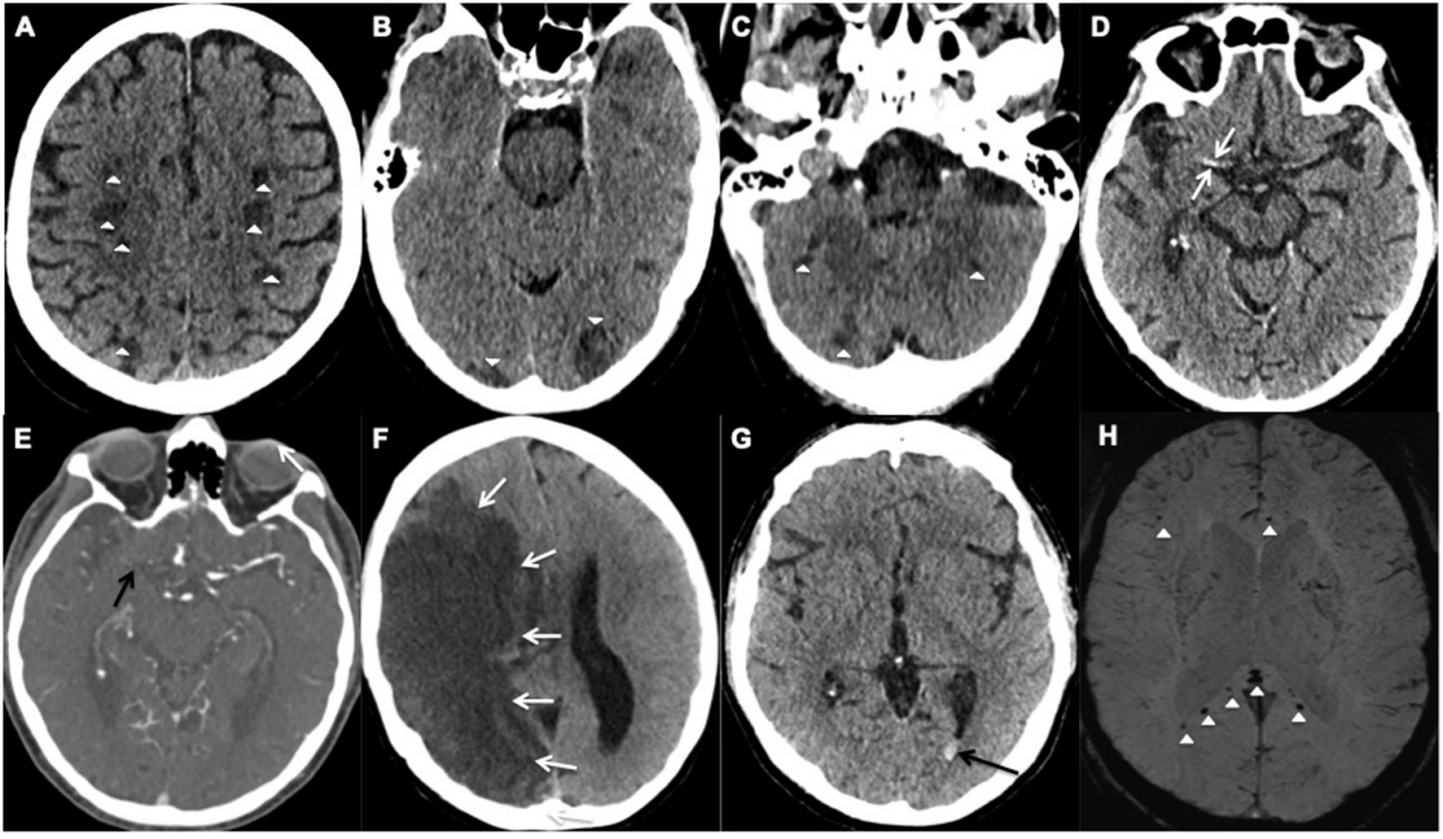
Representative examples of cerebrovascular events; (A–F) acute ischemic stroke and (G,H) hemorrhagic events. (A–C) Axial unenhanced CT images of Patient 28 demonstrate multifocal infarcts (white arrowheads) bilaterally along the (A) centrum semiovale, (B) Occipital lobes, and (C) Cerebellar hemispheres. (D,E) Axial CT images of Patient 25 demonstrates the (D) hyperdense right middle cerebral artery (MCA) sign (white arrows) and (E) corresponding filling defect on the CT angiogram confirming an occlusive thrombus (black arrow). (F) Axial unenhanced CT image of Patient 6 shows a large right MCA territory infarct (white arrows) with mass effect in keeping with an MCA malignant syndrome. (G,H) Axial images of Patient 18. (G) unenhanced CT demonstrates hyperdense layering in the occipital horn of the left lateral ventricle (black arrow) in keeping with isolated intraventricular hemorrhage (IVH), (H) Susceptibility weighted imaging confirms the IVH bleed within the left occipital horn (white arrow) and shows microbleeds at the splenium and genu of the corpus callosum, and subcortical white matter (white arrowheads).
